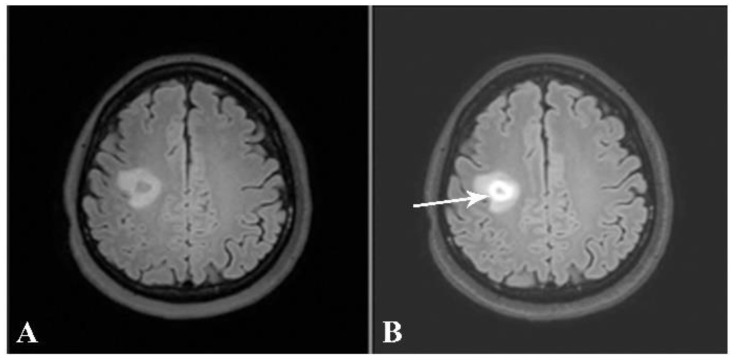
Axial precontrast FLAIR (A) and postcontrast FLAIR (B) images show complete ring-enhancing lesion (arrow) with minimal surrounding vasogenic edema. No significant pressure effect is shown. A presumptive diagnosis of small tumefactive MS was made, although there were no other lesions, either in the brain or in the spinal cord. The patient was given high-dose intravenous steroid.
